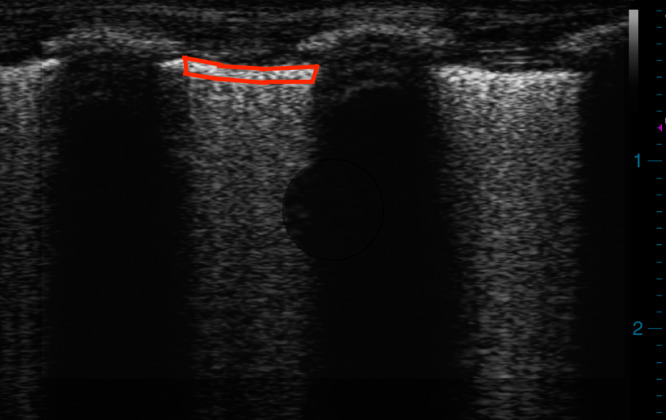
ROI (red tracing) for measurement of Q-LUS_MGV_. The ROI was delineated by (1) superior margin defined by the pleural surface; (2) lateral margin defined by the rib shadows; and (3) inferior margin defined by a depth of 50 pixels corresponding to one millimetre in depth. Q-LUS_MGV_; quantitative lung ultrasound mean grey value.
